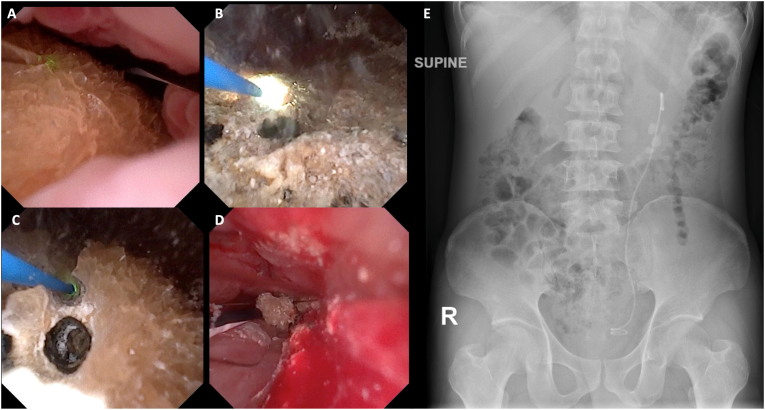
Intraoperative findings and postoperative outcome of RIRS in a horseshoe kidney. (A) Initial visualization of the renal stone before laser lithotripsy. (B-C) The progression of laser lithotripsy, showing the fragmentation of the stone. (D) Final intraoperative view showing the last remaining stone fragments before evacuation. (E) Postoperative plain abdominal radiograph (supine position) demonstrating correct placement of the left double-J stent with no visible residual radiopaque stones.
